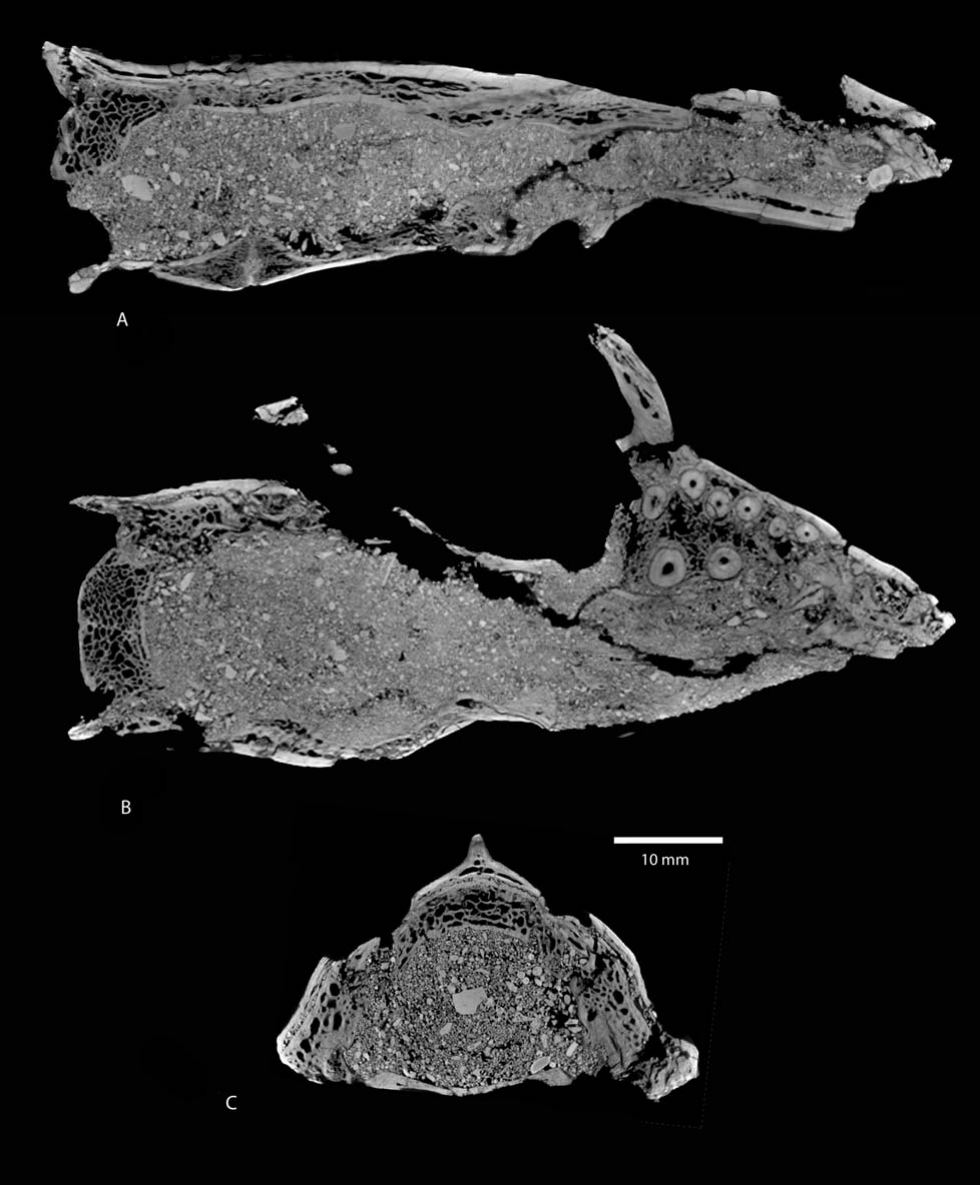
Ocepeia daouiensis*, Selandian, Phosphate level IIa of Sidi Chennane, Ouled Abdoun Basin, Morocco. CT scan sections of the skull MNHN.F PM45; A. Sagittal section YZ 299; C. Horizontal section B-XZ; C. Transverse section XY 704 B-XZ. Scale bar: 10 mm.
